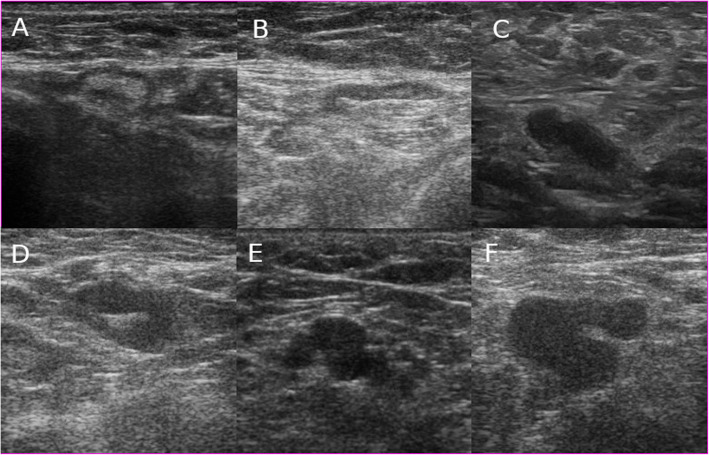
Axillary lymph node ultrasound images. Non-suspicious lymph node in clinically node negative cN0 patient and non-metastatic nodes confirmed after ALND (A). Axillary lymph node with eccentric thickening of the cortex in the US, positive FNAC, and one metastatic lymph node in the ALND (B). Atypical lymph node in the US in a clinically N1 patient, with positive FNAC and one metastatic node in the ALND (C). Atypical lymph node with uniform cortical thickening (> 3mm) in a cN0 patient with positive FNAC and more than three metastatic nodes in the ALND (D). Atypical lymph node with compressed fatty hilum and cortical thickening in a cN0 patient with positive FNAC and > 3 metastatic nodes in the ALND (E). Irregular node with a mass-like appearance in a cN0 patient, positive FNAC and > 3 metastatic nodes in the ALND (F)
